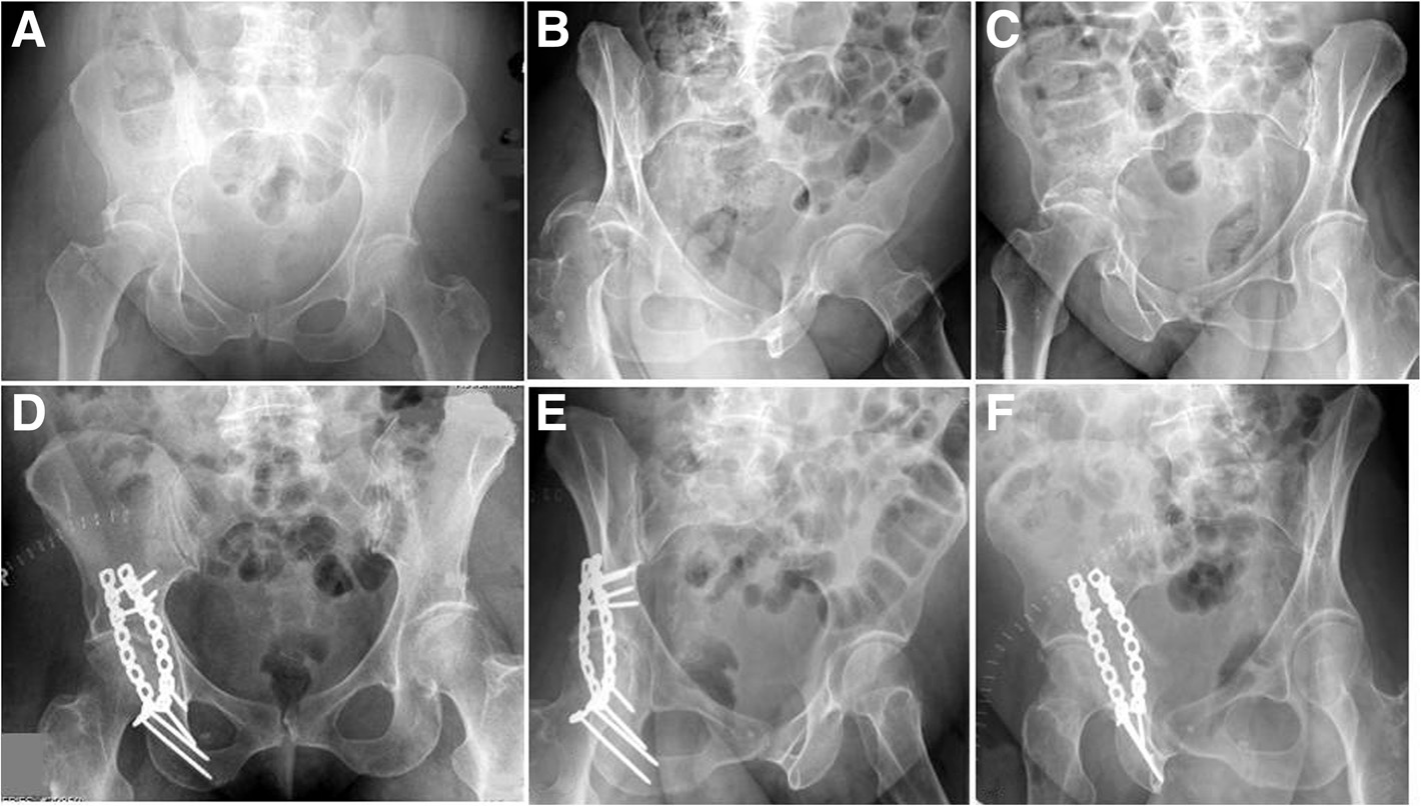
Example of an associated posterior column and posterior wall fracture treated with two 3.5 mm reconstruction plates. (A-C) Preoperative X-rays (a.p., obturator oblique, iliac oblique). (D-F) Postoperative X-rays (a.p., obturator oblique, iliac oblique).
